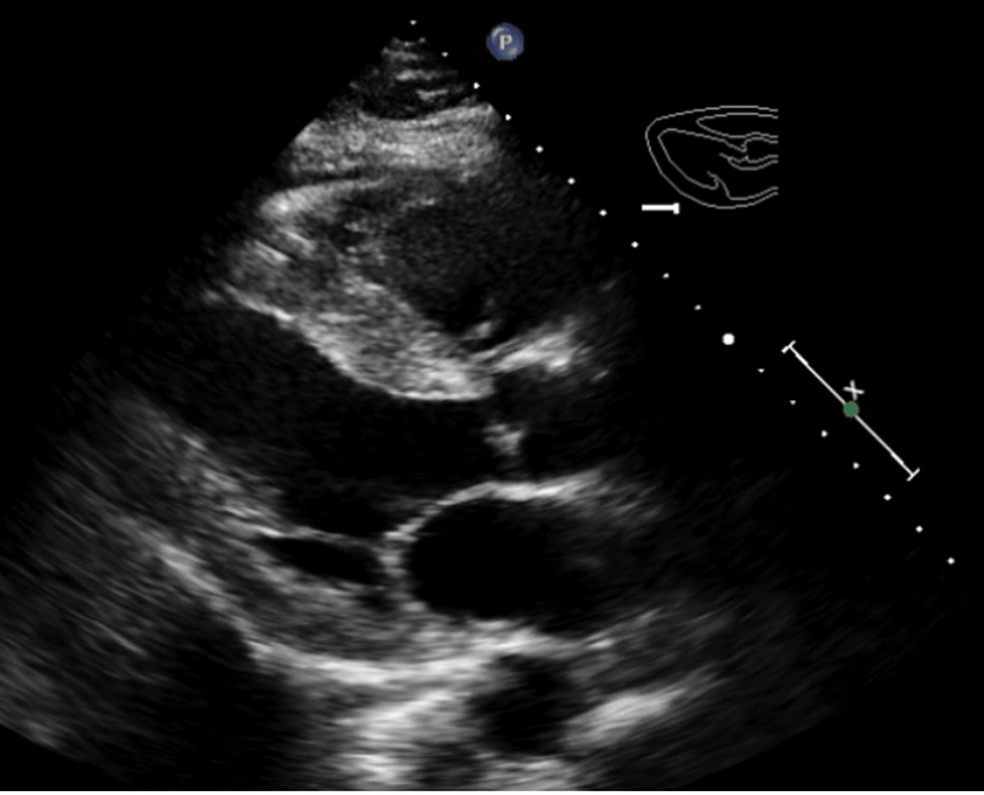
Transthoracic echocardiography revealed severe global hypokinesis and systolic dysfunction with an estimated left ventricular ejection fraction of 25-30%, mild concentric left ventricular hypertrophy, grade one diastolic dysfunction, and mild right ventricular dysfunction
